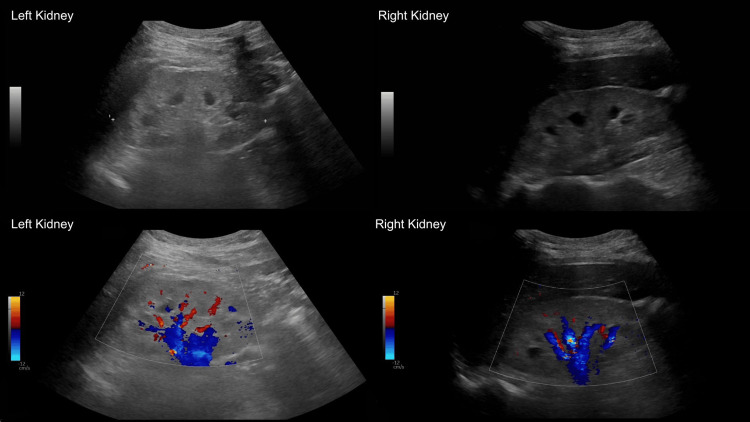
Renal ultrasound Ultrasound images of both kidneys demonstrate increased cortical echogenicity.
